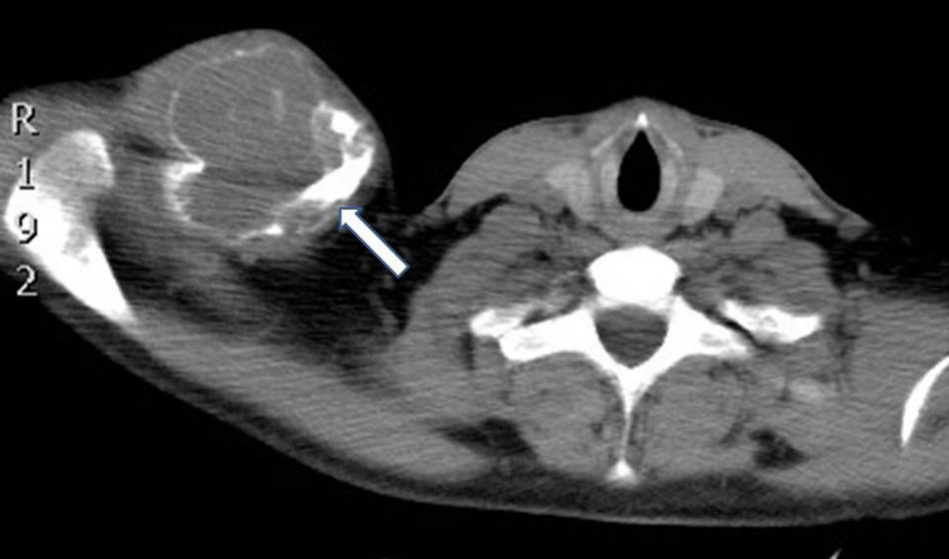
Case 1: CT scan of right clavicle tumor (arrow)
